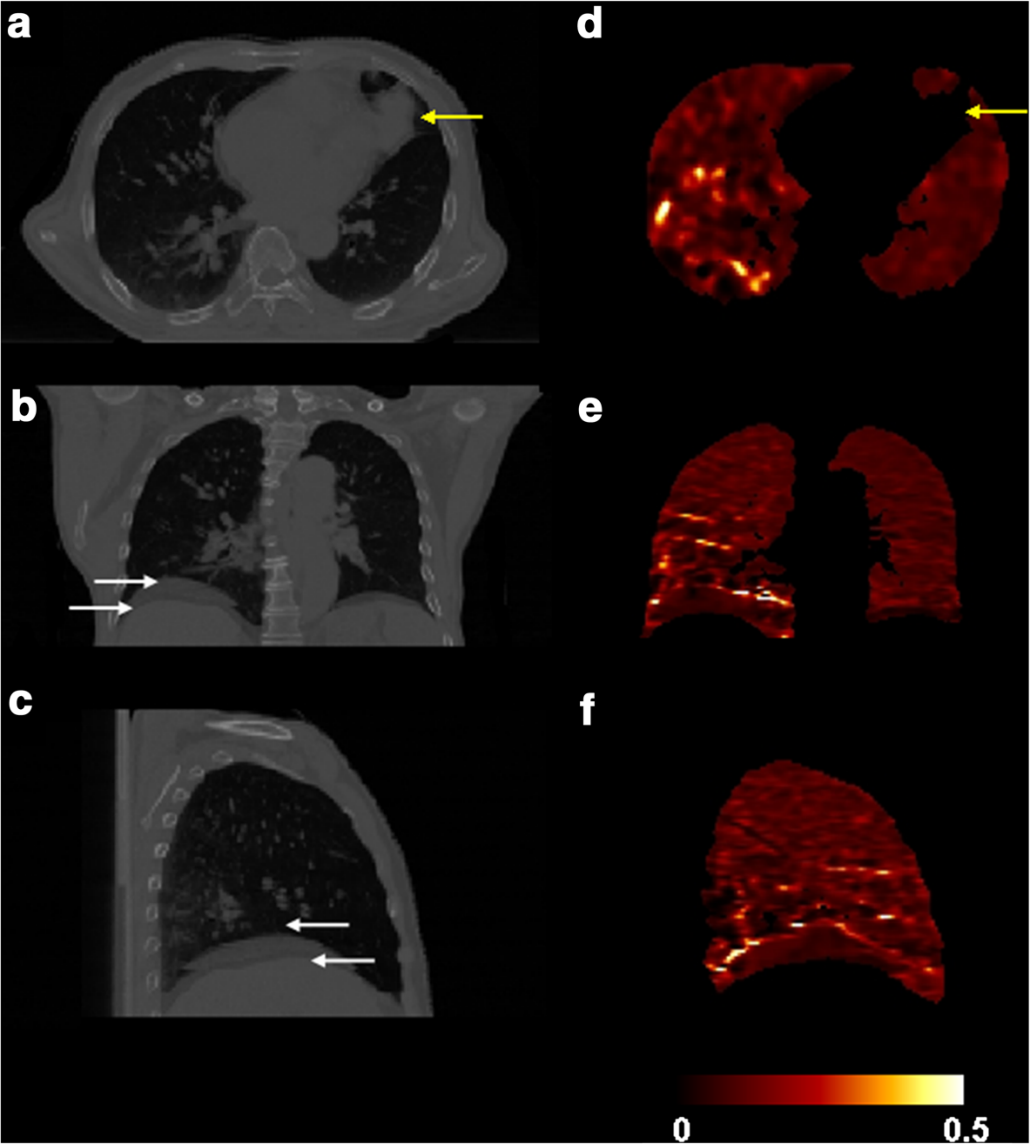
The transverse (a), coronal (b) and sagittal (c) views of a 4D-CT. The arrow indicated the location of the tumor. Corresponding views of 50%-0% (expiration-inspiration) ventilation of this example are shown as in (d), (e) and (f). Low ventilation is noticeable in the tumor region (indicated by the arrow on the CT image) on the ventilation image. The arrows on the CT image set indicate that the right diaphragm motion range was large. This volume change difference can be observed on the ventilation image. The ventilation image is scaled as *ΔV/V* = 0 as black and 0.5 as white.
